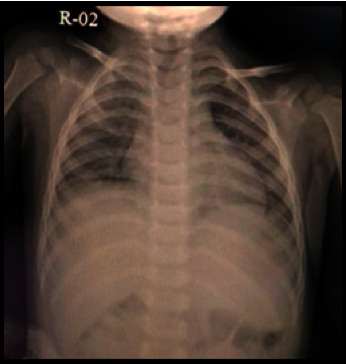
X-ray showing lung improvement.
